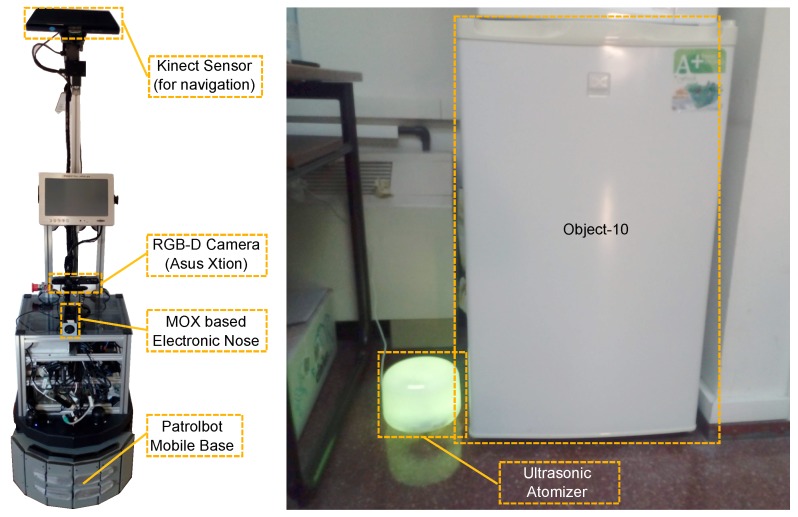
(Left) picture of the mobile robot employed during the real experiments. Besides the standard sensors used for navigation (2D lasers and Kinect), the robot was equipped with an additional RGB-D camera to perform object recognition, and a MOX-based e-nose [38] for gas detection and classification. (Right) photo of the ultrasonic atomizer releasing a mixture of water and ethanol next to the fridge (*Object-10*).
